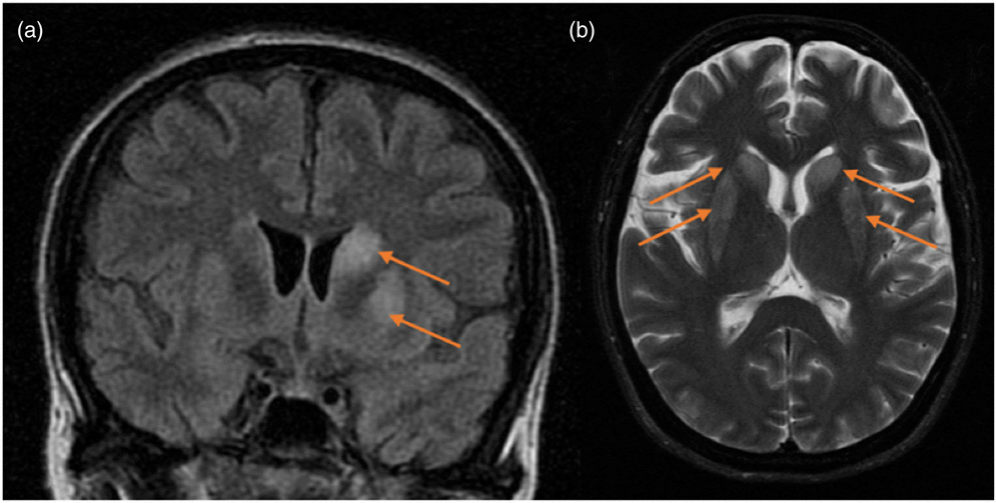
Diffuse hypoxic ischaemic encephalopathy. A 53-year-old female patient with primary pulmonary hypertension underwent double-LT. Figures: coronal MRI FLAIR sequence (a) and axial MRI T2-weighted sequence (b). The images show bilateral symmetrical hyperintensities and swelling in both caudate and putamina, left more than right (arrows).
